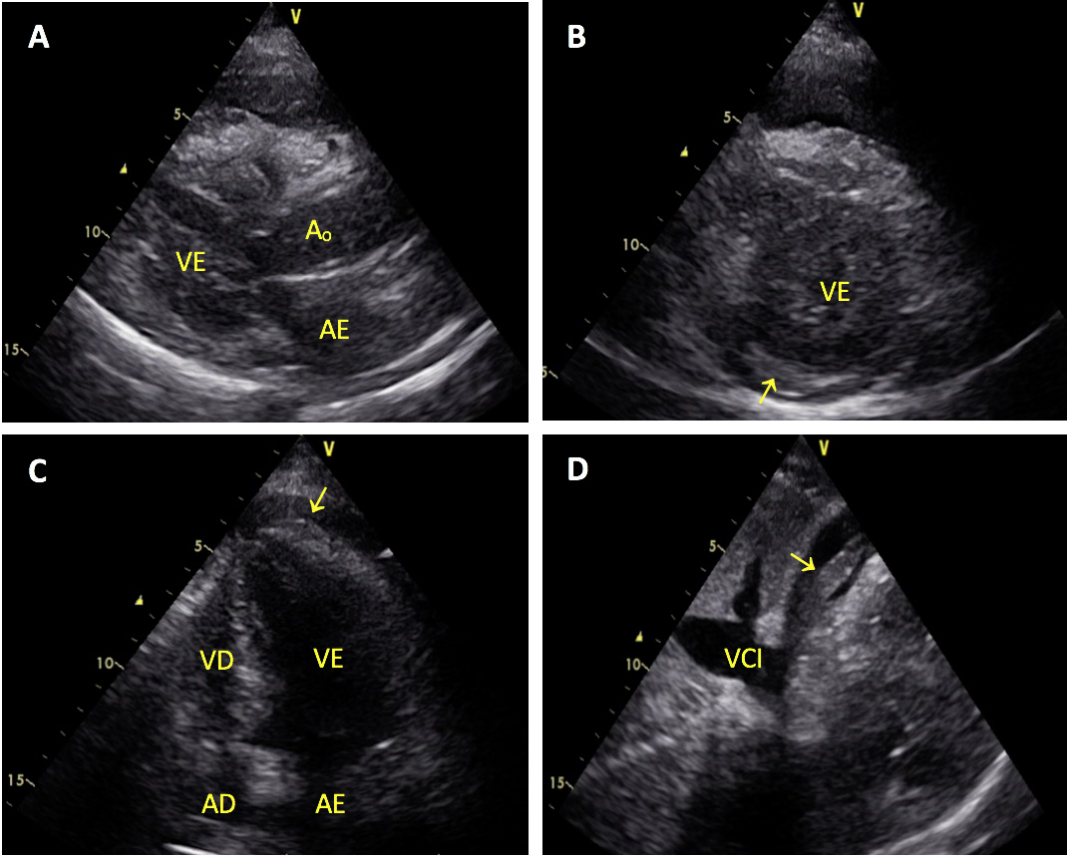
Ecocardiograma transtorácico. (A) Janela paraesternal longo eixo e (B) Janela paraesternal curto eixo mostrando um ventrículo esquerdo ligeiramente hipertrofiado e não dilatado; (C) Janela apical 4 câmaras mostrando colapso parcial das cavidades direitas e hipocinesia da parede lateral, mas função sistólica do ventrículo esquerdo preservada; (D) Janela subcostal mostrando veia cava inferior dilatada, sem variação respiratória. A seta amarela indica derrame pericárdico moderado. VE: ventrículo esquerdo; AE: átrio esquerdo; Ao; aorta ascendente; VD: ventrículo direito; AD: átrio direito; VCI: veia cava inferior.
